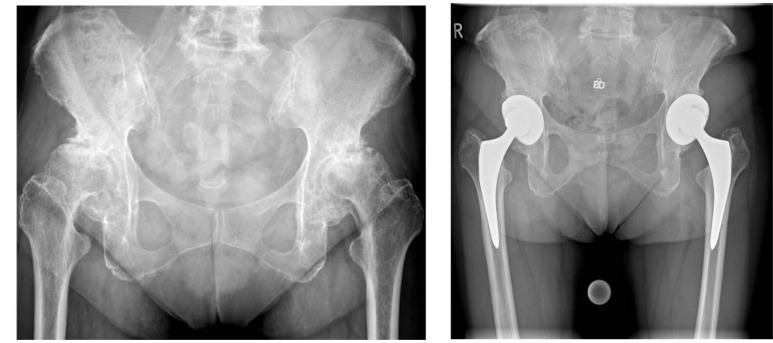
A 69 year old female patient. a) Preoperative anteroposterior radiograph, showing bilateral osteoarthritis. b) Immediate postoperative radiograph. Correct position of the implants.
